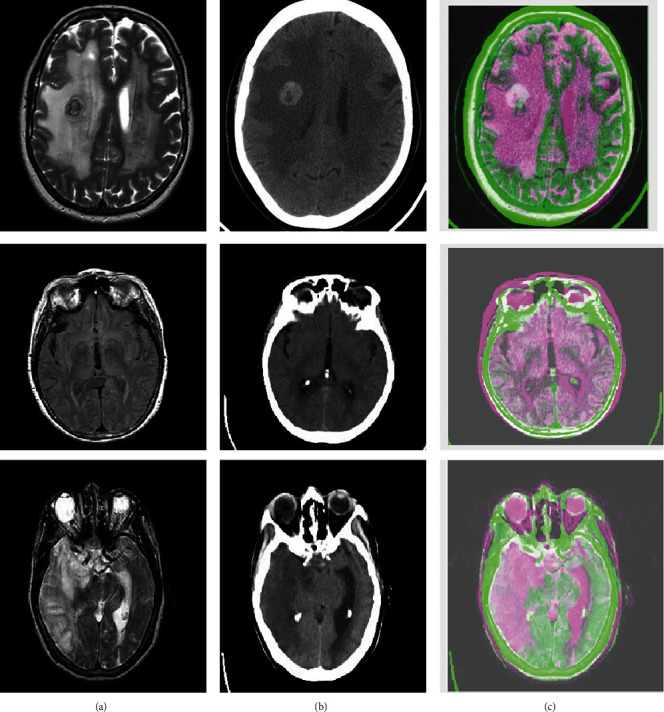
MRI and CT Brain of size 256 × 256 pixels and its fused images. (a) Input-MRI image. (b) Input-CT image. (c) Fused MRI-CT image by using the average method.
